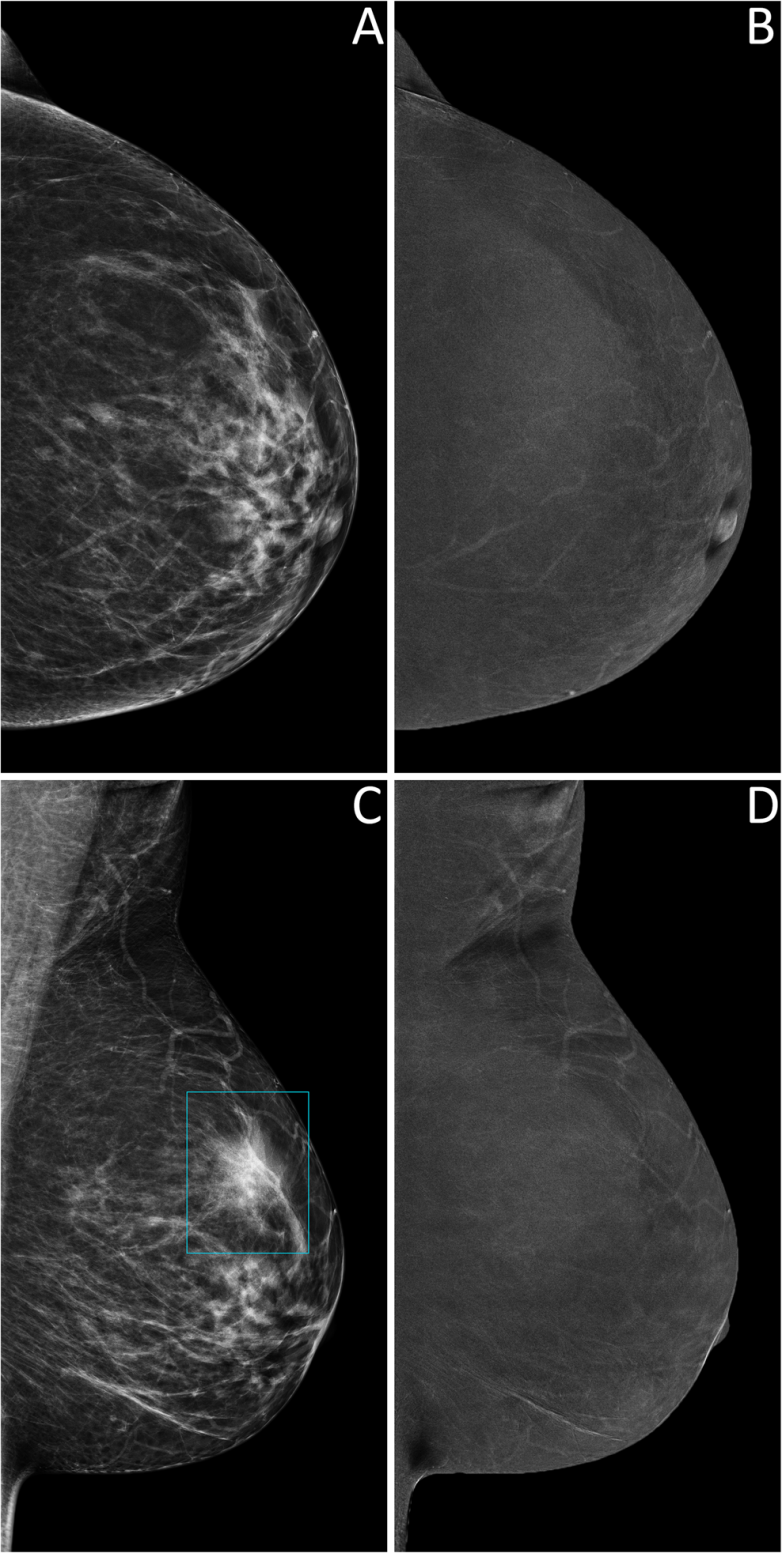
True negative case at contrast-enhanced mammography. A 49-year-old woman was recalled for a suspicious asymmetry in the upper quadrants of the left breast, not observable on the craniocaudal view (low-energy image, panel A) but definitely noticeable on the mediolateral oblique view (low-energy image, panel C, light blue rectangle). Standard assessment referred this finding to ultrasound-guided core needle biopsy, leading to a diagnosis of fibrosis. Conversely, the absence of enhancement in recombined images, both on the whole craniocaudal view (panel B) and in correspondence of the suspicious area on the mediolateral oblique view (panel D) would have oriented the work-up to a normal result with referral to re-screening at a 2-year interval
